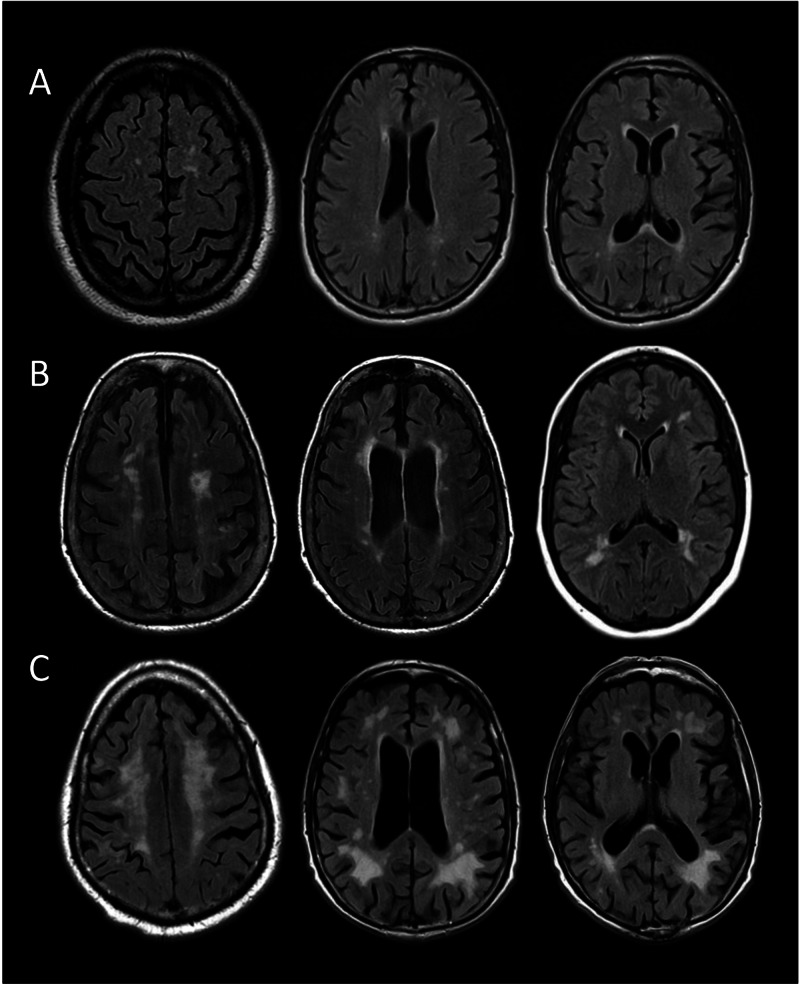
Grading of leukoaraiosis severity based on the Fazekas scale. (A) Fazekas grade 1 (mild) with periventricular caps of the ventricles or punctate foci in the deep white matter; (B) Fazekas grade 2 (moderate) with smooth periventricular halo or convergence of deep white matter lesions; (C) Fazekas grade 3 (severe) with confluent periventricular leukoaraiosis extending into the subcortical deep white matter. MRI examples from actual patient population. MRI = magnetic resonance imaging
